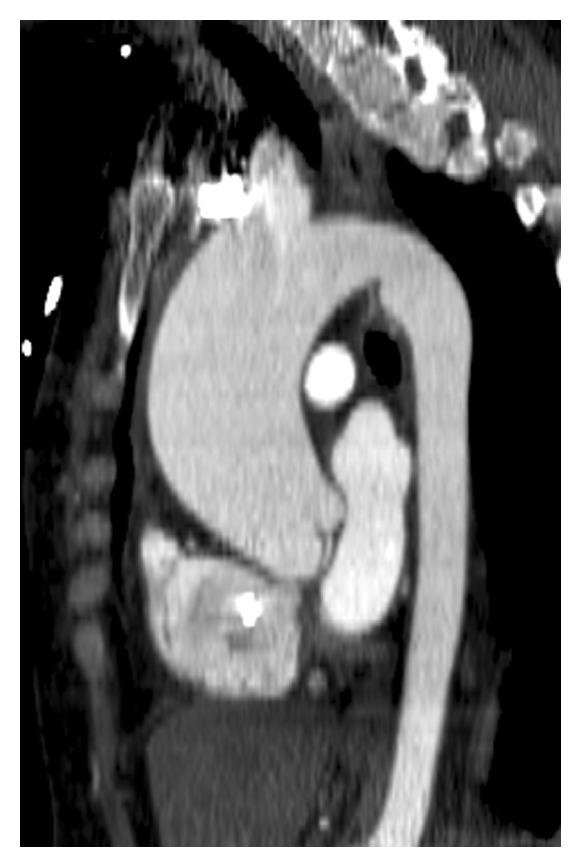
Fusiform ascending aortic aneurysm—sagittal reconstructed CT image in a 47-year-old male shows a smooth, fusiform aneurysm of the ascending aorta, which tapers to normal caliber at the proximal arch.
